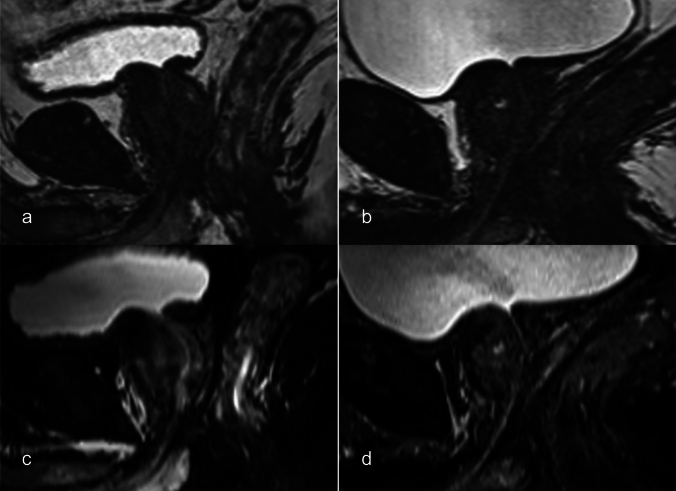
Sagittal images of a 76-year-old male in Case 2 (a, c). The DSC value of the conventional 3D T2-weighted image was 0.64 and the visibility score was 2, whereas the DSC value of the proposed fat-suppressed 3D T2-weighted image was 0.81, and the visibility score was 4. Sagittal images of a 67-year-old male in Case 4 (b, d). The DSC value of the conventional 3D T2-weighted image was 0.76, and the visibility score was 3, whereas the DSC value of the proposed fat-suppressed 3D T2-weighted image was 0.87, and the visibility score was 4
